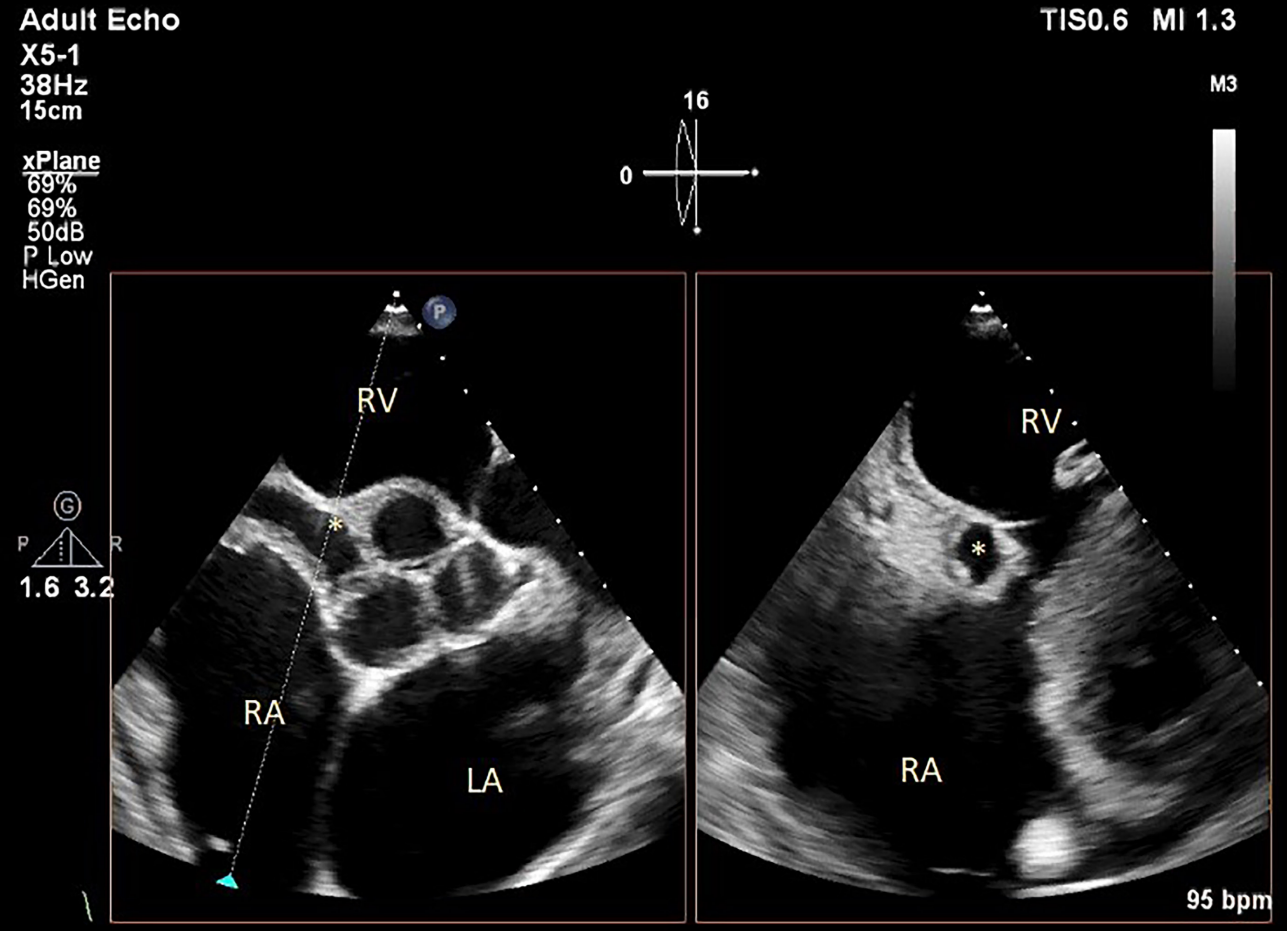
Transthoracic echocardiography using the biplane method shows a tunnel shape VSD (*). LA: left atrium; RA: right atrium; RV: right ventricle.
